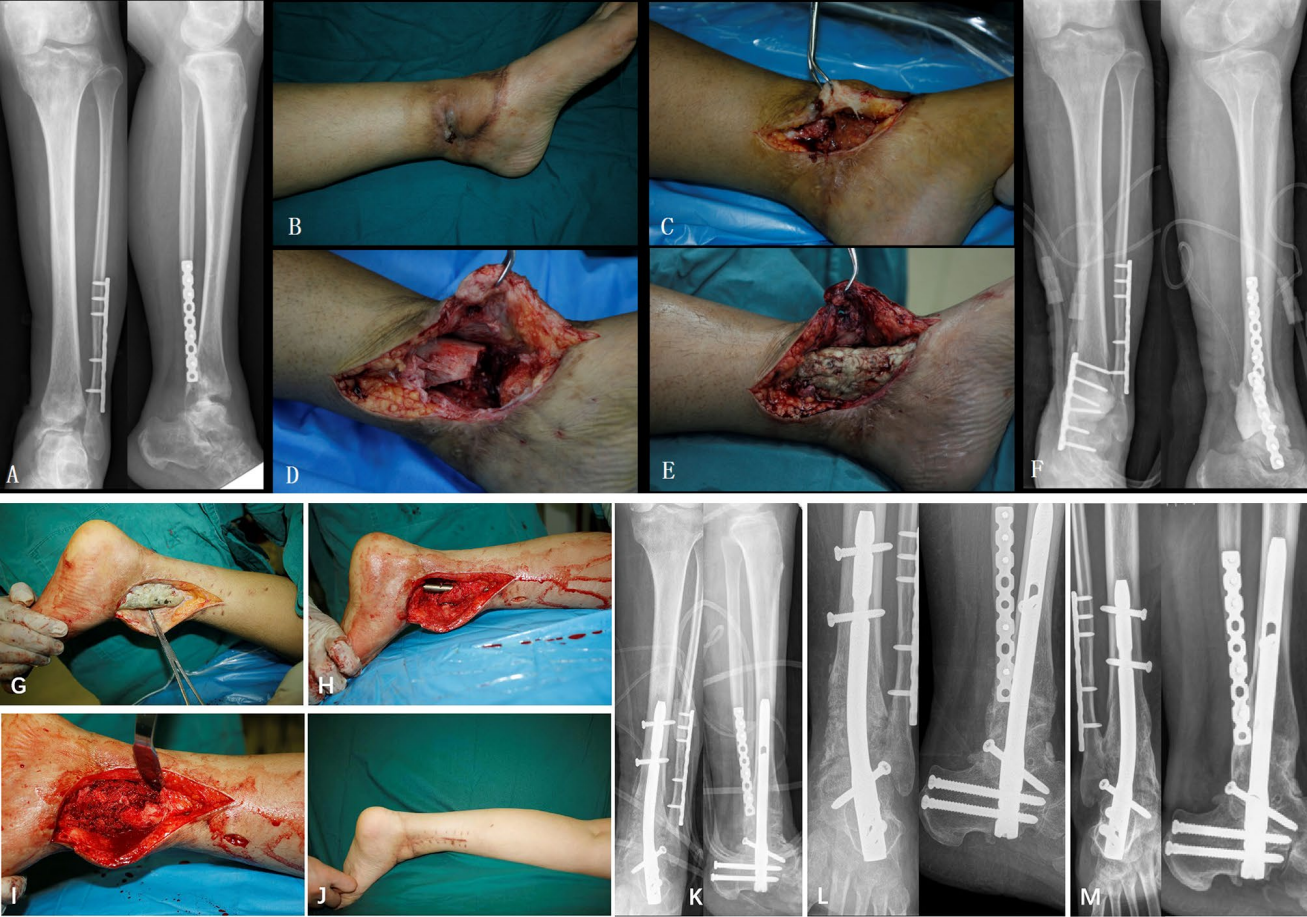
A 29-year-old female presented with repeated skin ulceration and pus of the left leg for 8 months. (A) Preoperative X-ray; (B) preoperative photograph; (C) pustules and necrotic tissue; (D) bone defects after debridement; (E) plate fixation and cement implantation; (F) postoperative X-ray; (G) pregrafting photograph showing no necrotic tissue; (H) retrograde intramedullary nail for ankle fusion; I: bone grafting; J: no ulceration or pus at the 3-month follow-up; (K) postoperative X-ray; (L) X-ray showing bone union occurring 6 months later; (M) bone union after 24 months.
